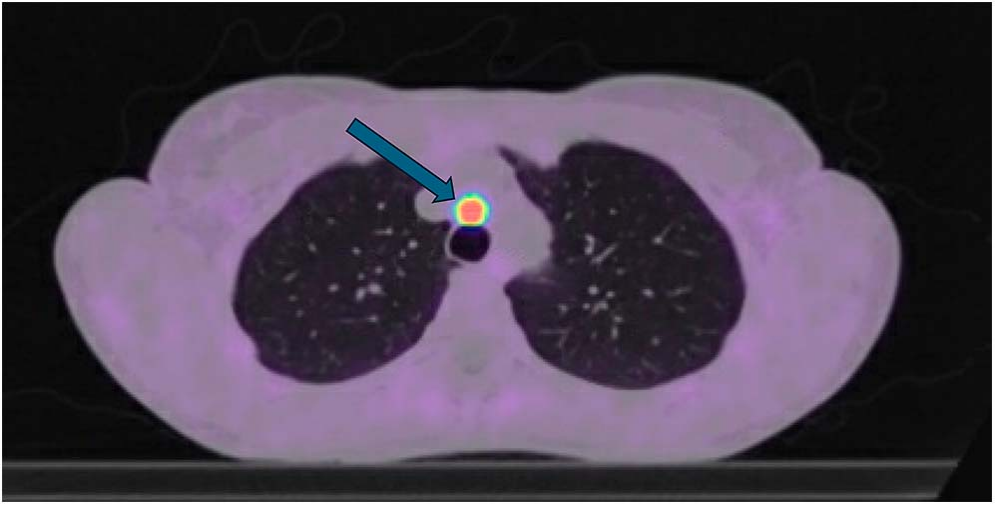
An image from a ^68^Ga-DOTATATE PET CT scan demonstrating a 6 mm anterior mediastinal PGL (arrowed). This lesion was not previously evident on MRI scanning.
